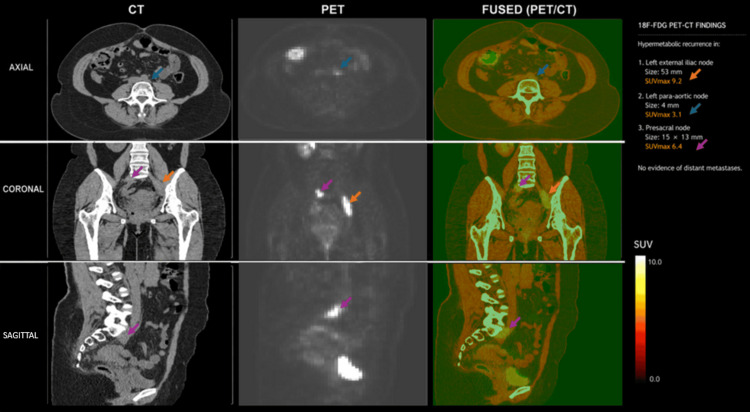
Diagnostic PET-CT of Patient 1 showing pelvic and para-aortic nodal recurrence. Hypermetabolic left external iliac (orange arrow), para-aortic (blue arrow), and presacral (purple arrow) lymph nodes were identified without evidence of distant disease. PET-CT: positron emission tomography-computed tomography; SUVmax: maximum standardized uptake value; ^18^F-FDG: fluorine-18 fluorodeoxyglucose
